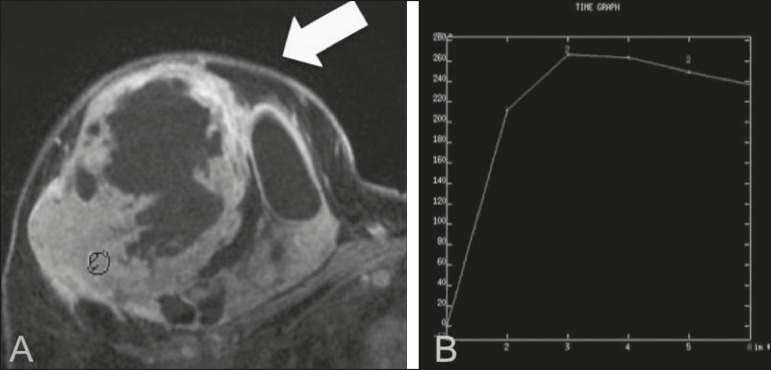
A: MRI showing a huge irregular mass with peripheral
